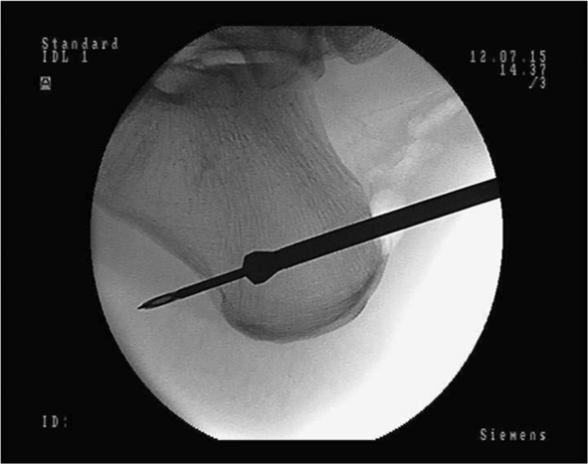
X-ray control of calcaneus drilling
